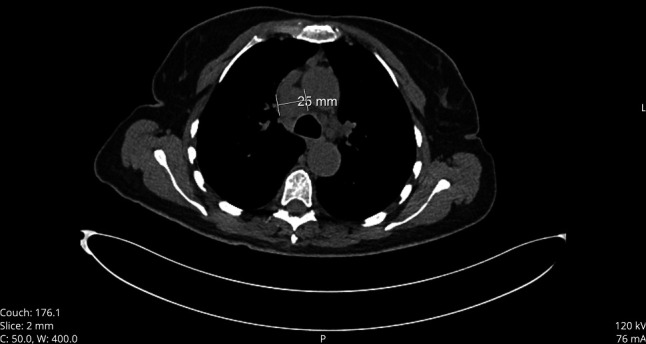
Chest CT showing paratracheal lymphadenopathy. Chest CT from December 14, 2023. CT, computed tomography.
